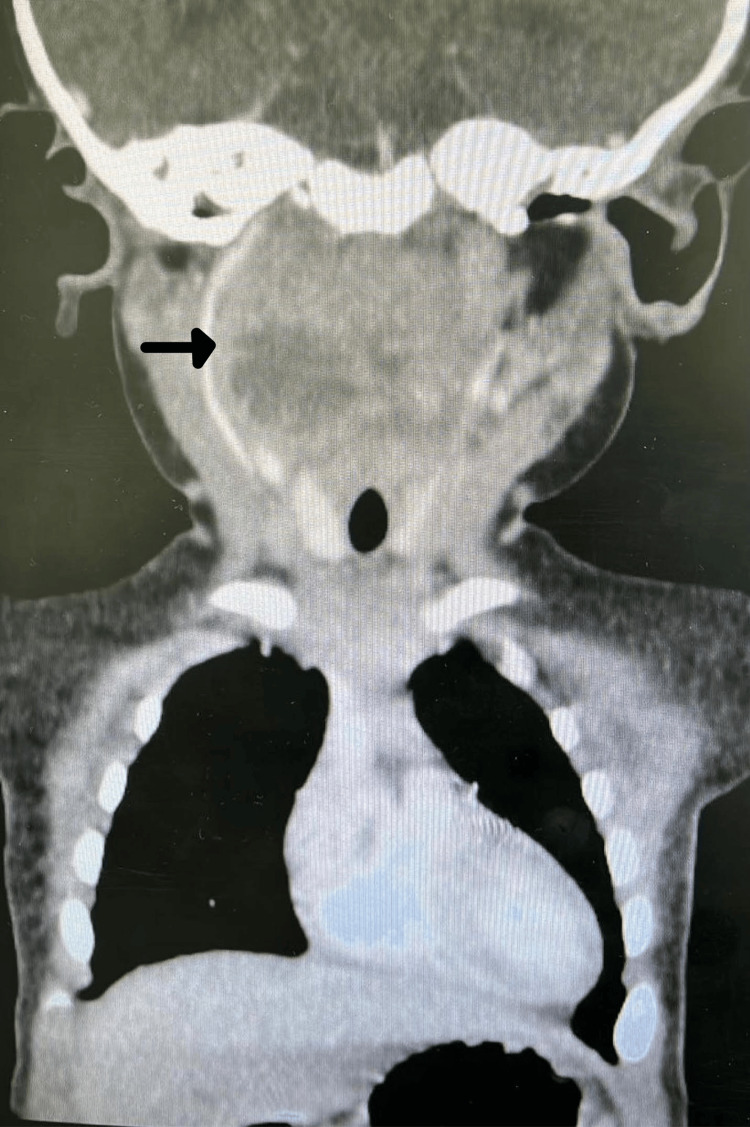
Coronal view of CT scan showing right-sided retropharyngeal and parapharyngeal abscess measuring about 27 x 33 x 12 mm in maximum dimensions that connects to the right side of oropharynx at C3 level with marked compromise of airway and multiple enlarged lymph nodes. CT: computed tomography; C3: cervical spine 3.
